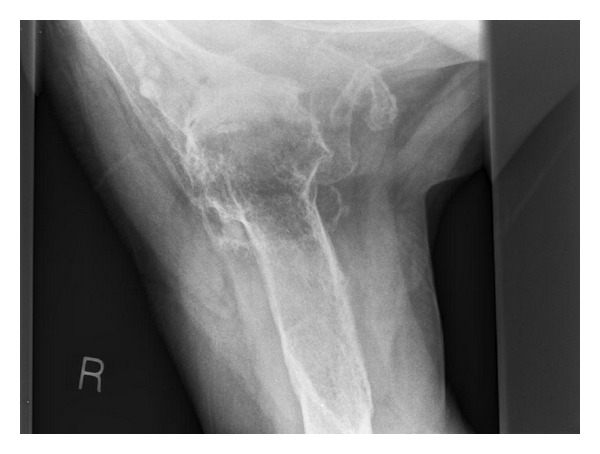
Axillary lateral radiograph of the right shoulder taken pre-operatively.
